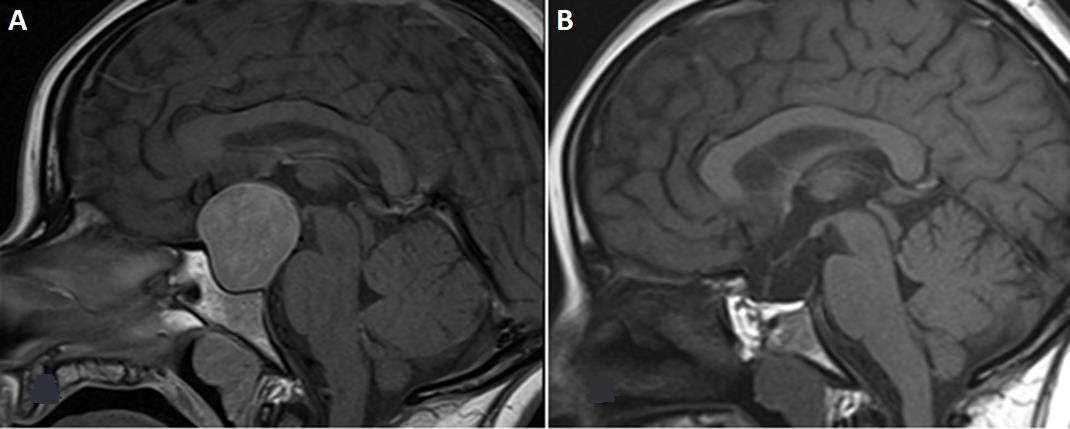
A) sagittal T1-weighted images preoperatively; (B) and postoperatively demonstrate the absence of residual tumor; sagittal T1-weighted images postoperatively reveal optic tract decompression, with the posterior pituitary bright spot clearly visible
